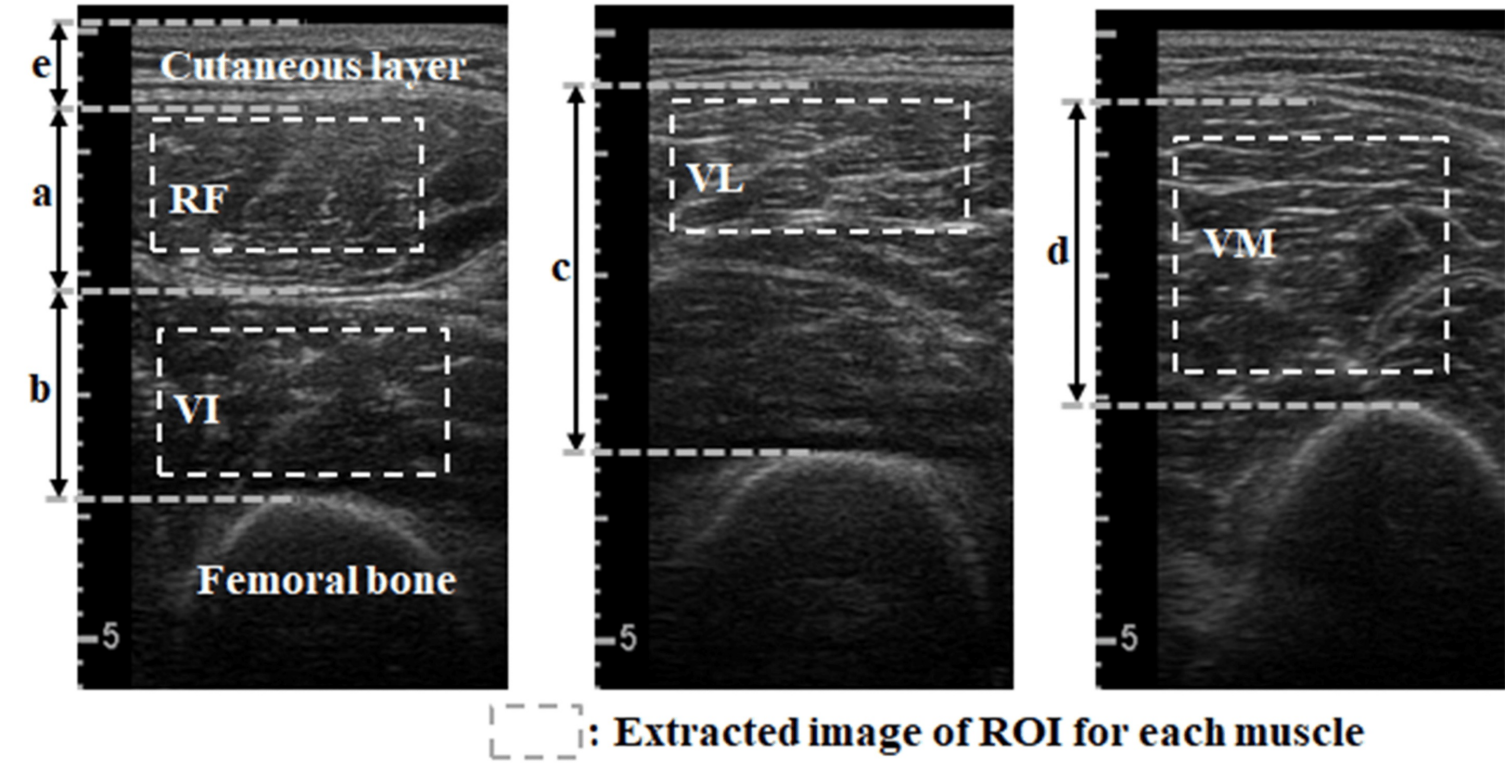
Ultrasound imaging of the quadriceps femoris to measure muscle and cutaneous thicknesses and echo intensities. The mean of grayscale values is considered the echo intensity. A: RF thickness; B: VI thickness; C: VL thickness; D: VM thickness; E: cutaneous thickness Abbreviations: RF, rectus femoris; ROI, region of interest; VI, vastus intermedius; VL, vastus lateralis; VM, vastus medialis
